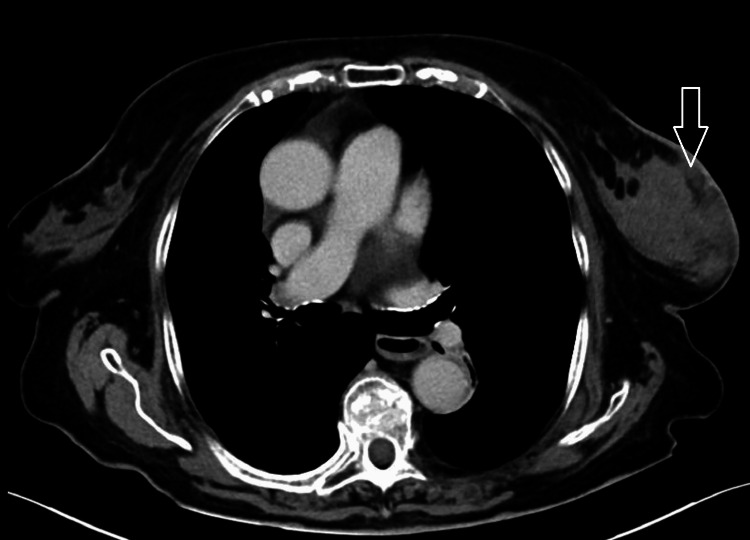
CT scan of the chest showing an infiltrative breast mass, without evidence of disease in other thoracic structures
